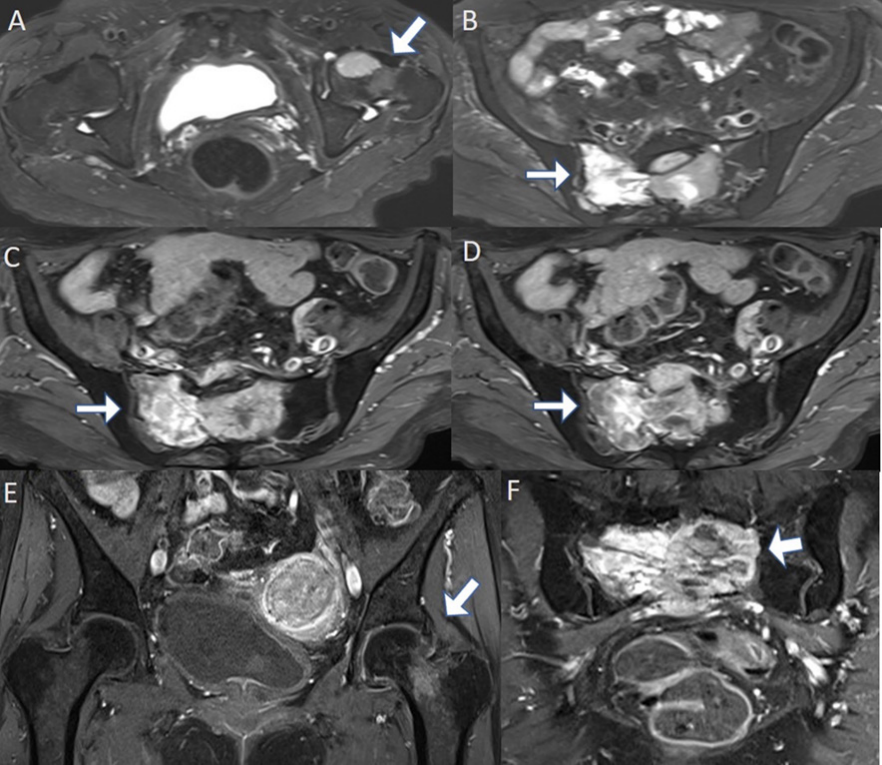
Axial *T*_2_W fat saturated (A, B) and axial (C, D) and coronal (E, F) *T*_1_W post-contrast (fat saturated) MR images of the pelvis show *T*_2_ hyperintense and enhancing tumour involving the left femoral neck and sacrum (arrows). These are consistent with osseous metastases of primary CNS haemangiopericytoma. CNS, central nervous system
